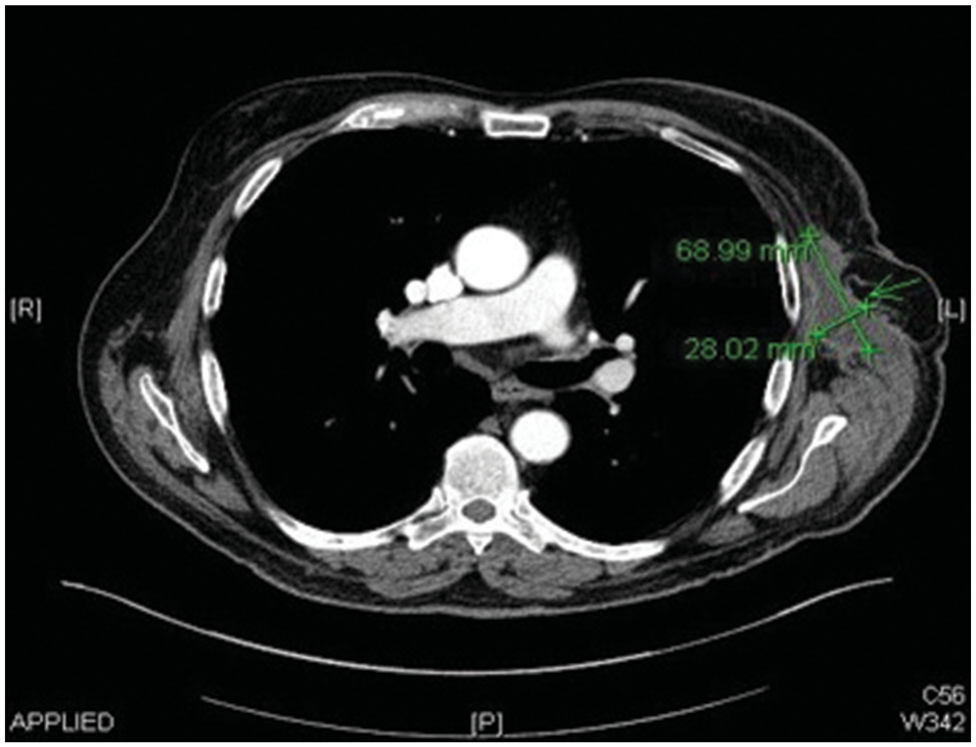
CT scan image 3-month post-resection of chronic seroma capsule showing almost complete resolution.
